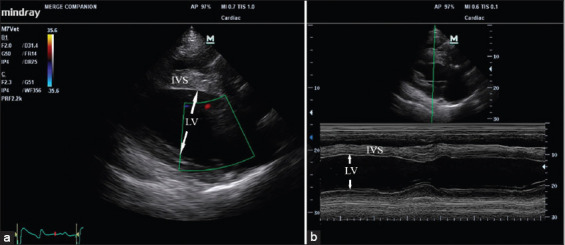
Echocardiographic of longitudinal image of heart (a) and M mode image of the left ventricle (b), arrow represented the left ventricular internal diameter; LV=left ventricle; IVS=Interventricular septum.
